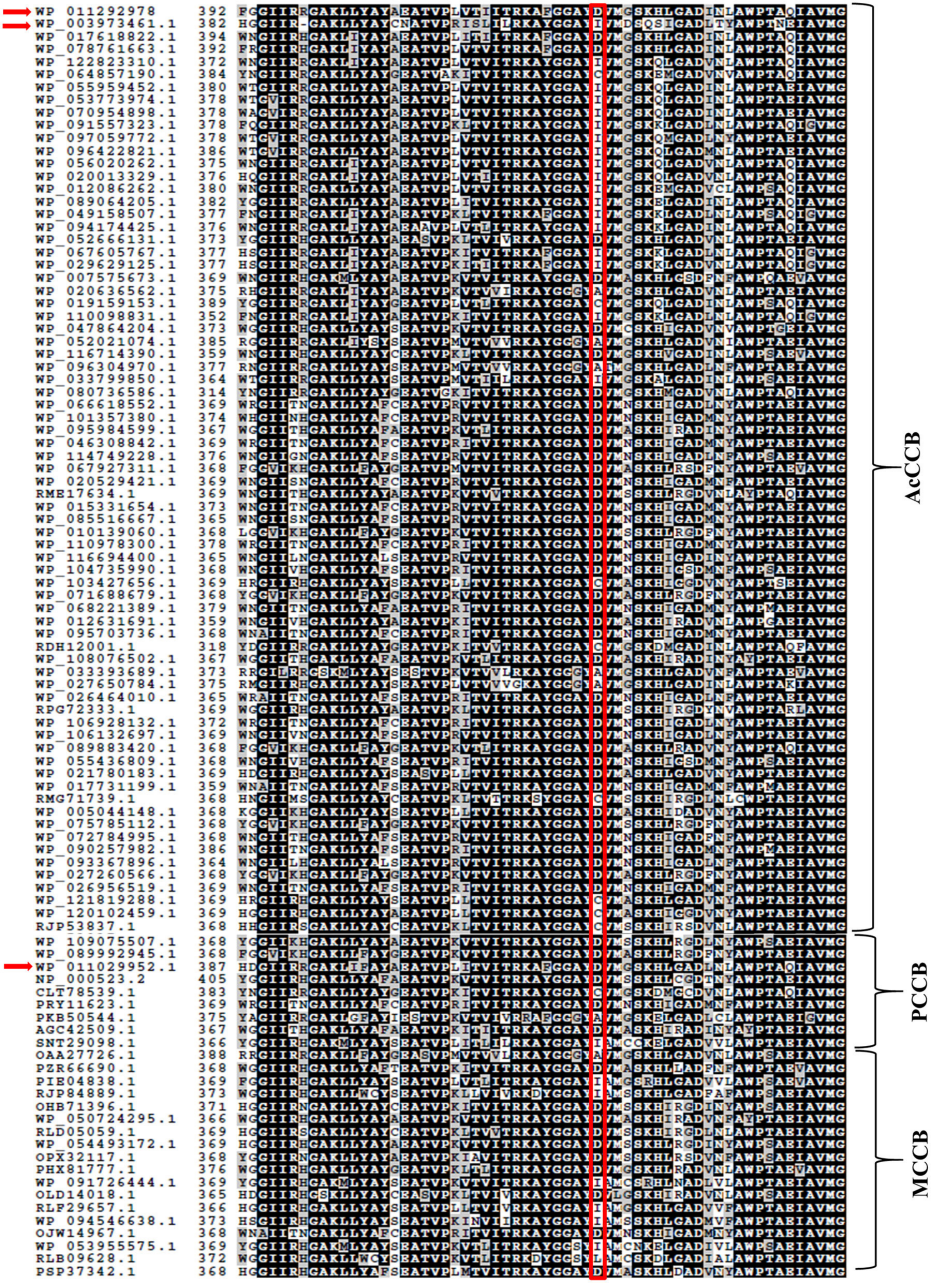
Amino acid sequence comparisons of CT subunits of AcCCase, PCCase and 3-methylcrotonyl-CoA carboxylase (MCCase) enzymes. Highlighted is the conserved Asp residue, near the C-terminus of these proteins that may play a role in determining the substrate specificity of these enzymes. The sequences of TfAcCCB (WP_011292978), ScAcCCB (WP_003973461.1), and ScPccB (WP_011029952.1) subunits are arrowed.
